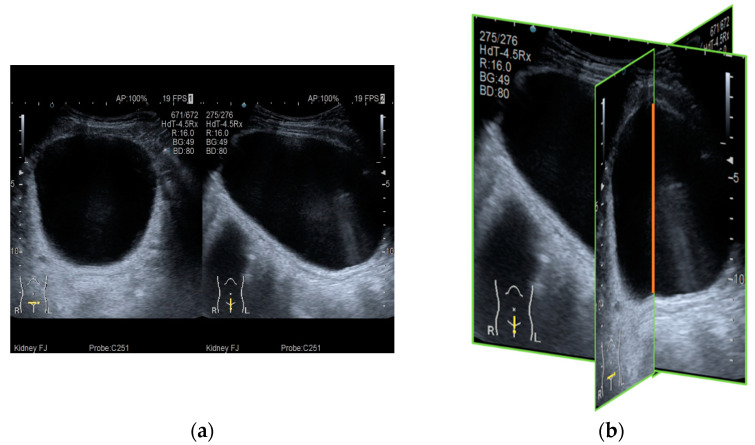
(a) Original 2D ultrasonic image. (b) The transverse view and sagittal view are aligned (crossover) in the maximum vertical line.
